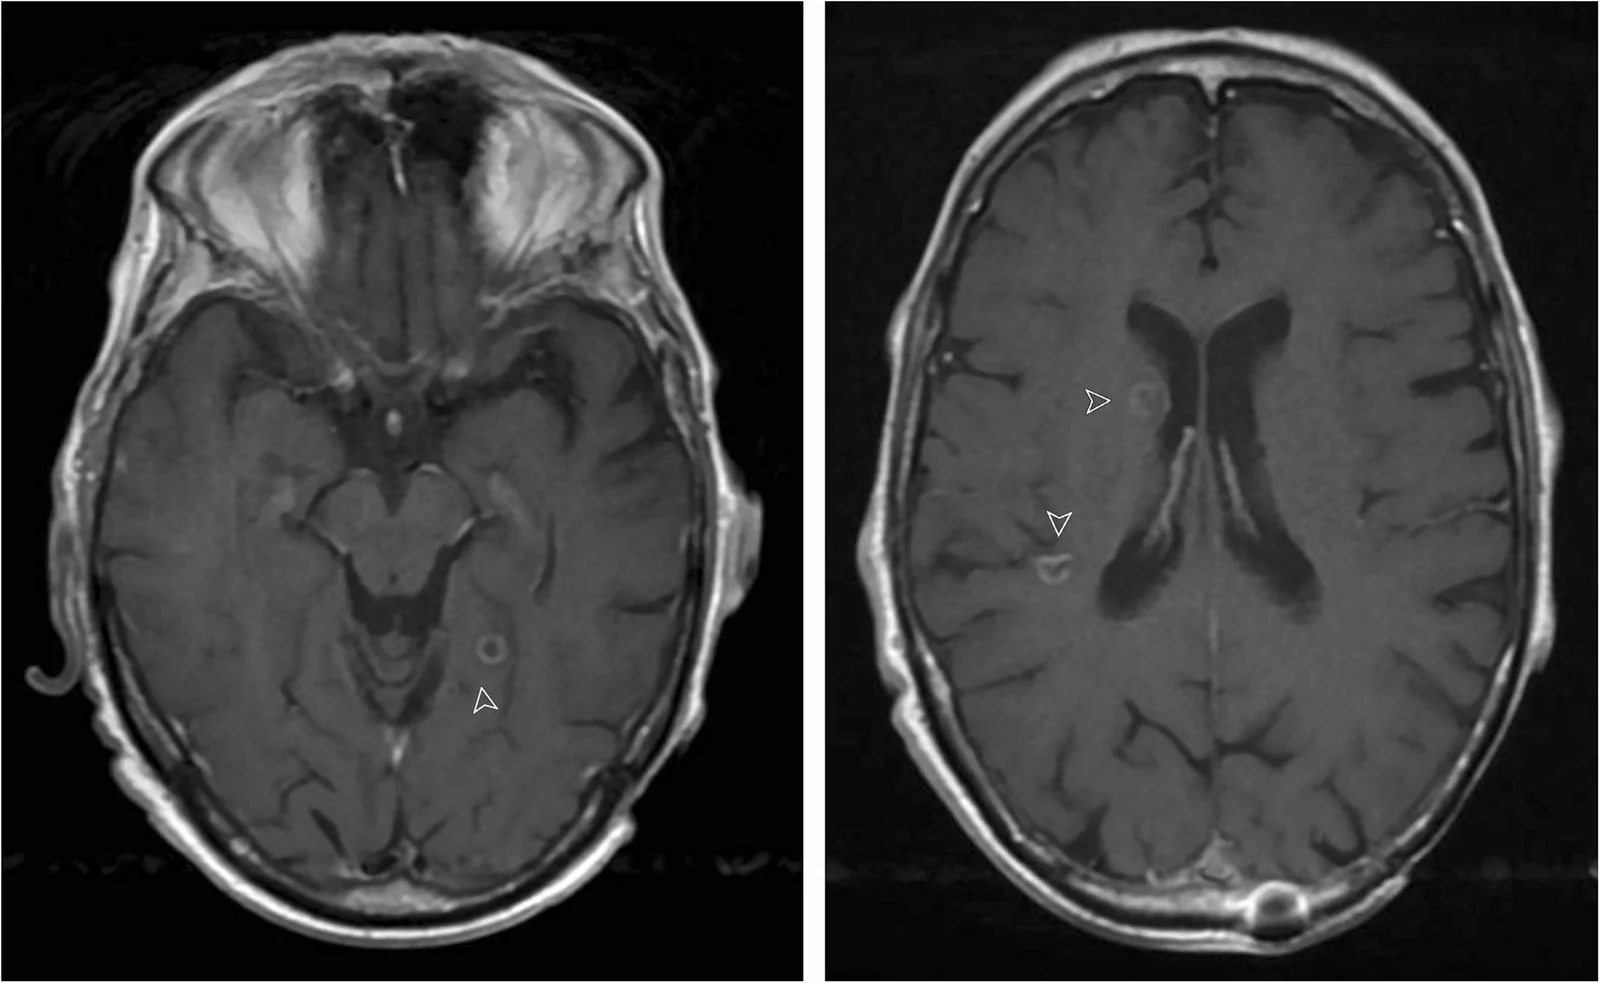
MRI brain (contrast enhanced T1 weighted) showing multiple rim enhancing foci within the basal ganglia (marked with white outlined arrows) with diffusion restriction in bilateral cerebral hemispheres.
